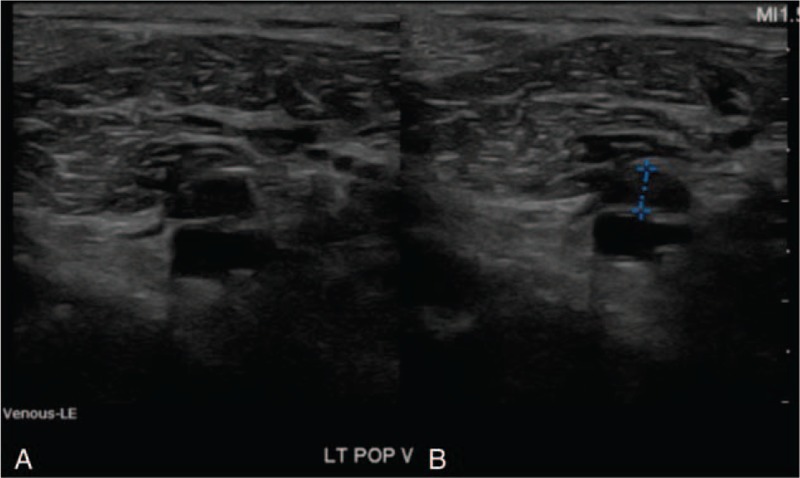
Color Doppler ultrasonography images of patient's left popliteal vein. (A) Popliteal vein and artery without compression; (B) Popliteal vein and artery with compression. Left popliteal vein is not compressible due to thrombus.
